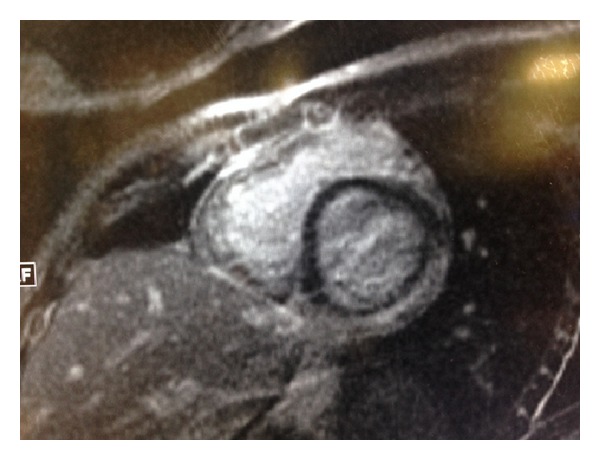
Cardiac MRI showing multiple foci of curvilinear and oval shaped contrast hyperenhancement involving the central portions of the anterior, lateral, and inferior wall of LV with relative sparing of the endocardial portions of the myocardial walls.
